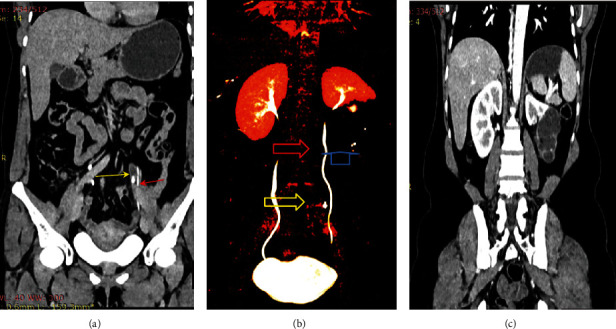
Abdominal CT scan showed the following: (a) coronal view shows both functioning (red arrow) and dilated ureter with proximal dilatation (yellow arrow); (b) 3D CT construction showing midureteric stone (yellow arrow), nonobstructed ureter with complete urinary drainage from upper segment (red arrow), and hydronephrotic segment (blue arrow); (c) contrast film showing lower pole nonfunctioning and hydronephrotic kidney.
